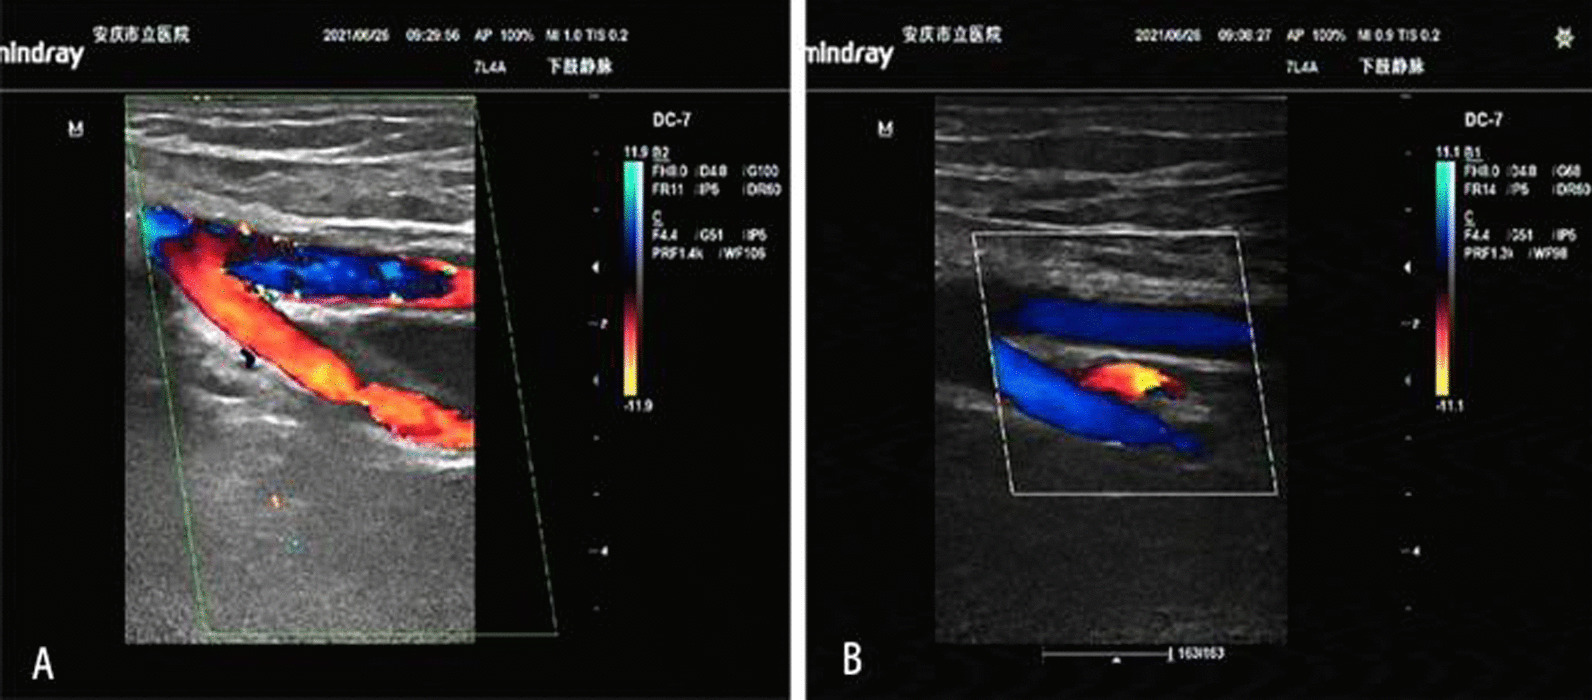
A, B No tumor thrombus was found by ultrasonography after chemoradiotherapy.
